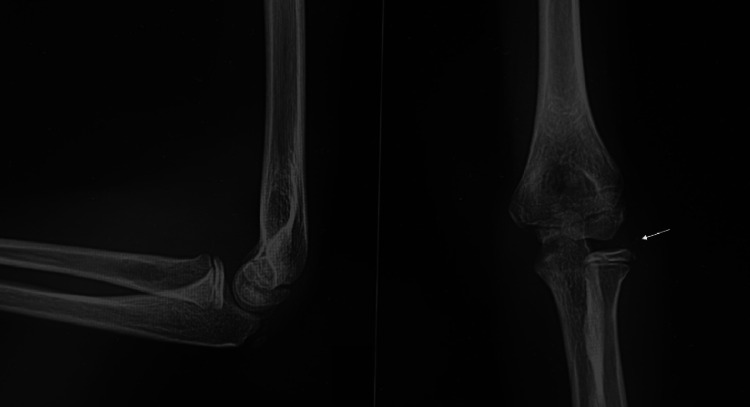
Left elbow radiographs: lateral and anteroposterior views Bony fragments in the radiocapitellar joint (white arrow).
